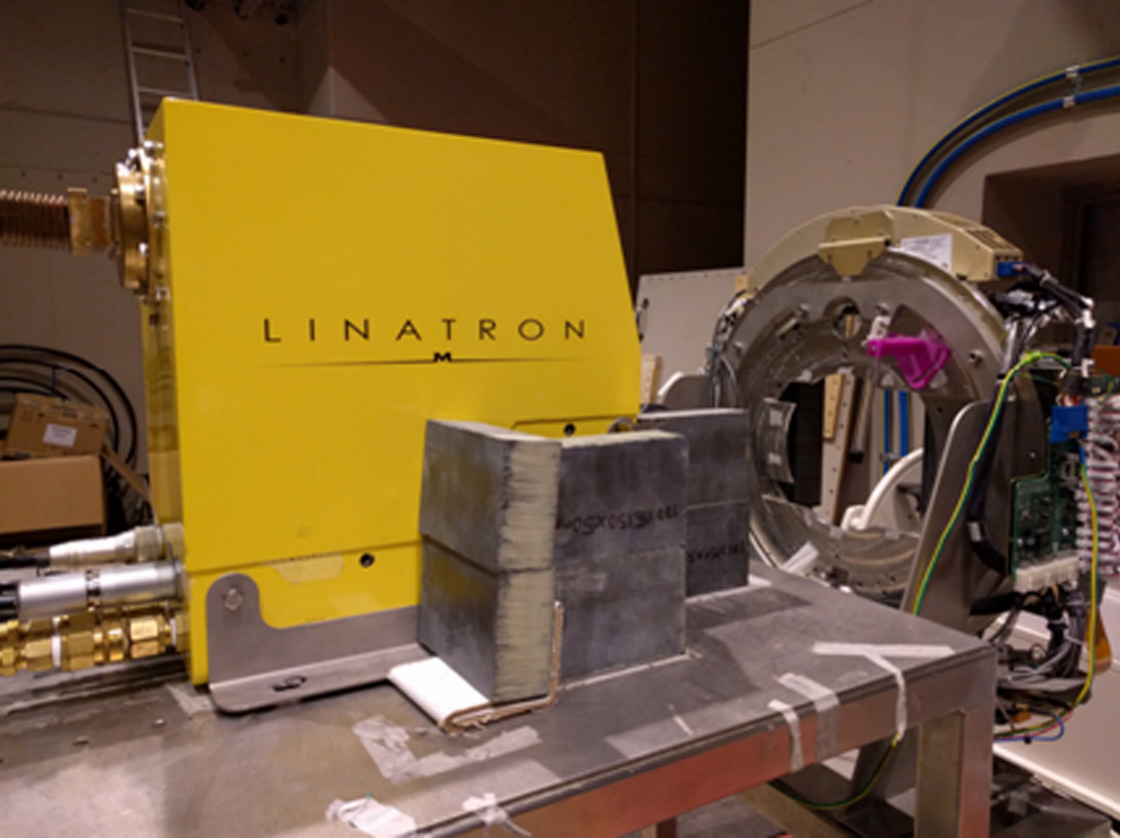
Fixed gantry linatron with multileaf collimators (MLCs) (white arrow) outside the magnetic resonance imaging faraday cage. The bore is positioned that the main magnetic field is aligned parallel with linatron beam.
